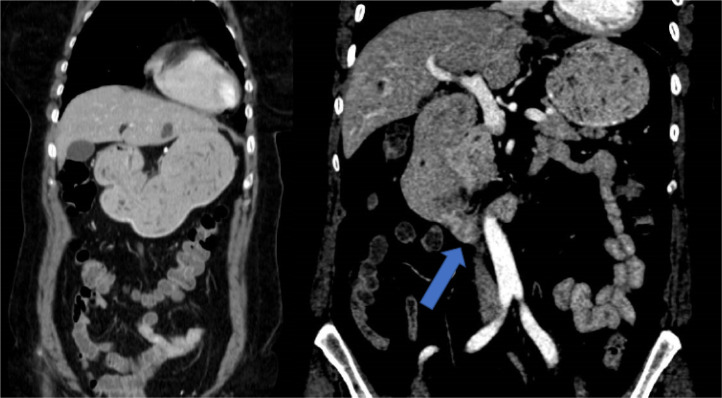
Urgent CT scan showed pseudonodular hypodense image depending on the lateral wall of the second duodenal portion (blue arrow) that causes distension of the gastric chamber
